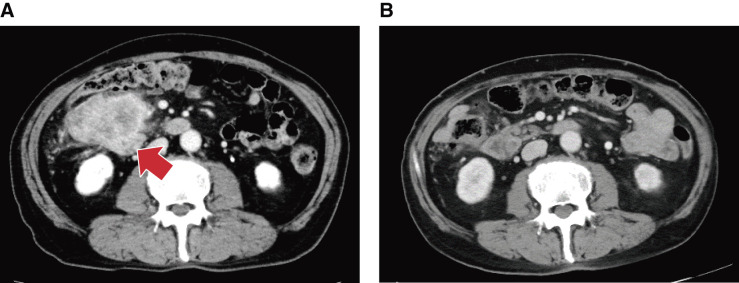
Comparison of pre- and post-immunotherapy on CT scan. (A) Duodenal invasion by the ascending colon cancer. The descending part of the duodenum is at the tip of the red arrow. (B) The tumor has shrunk significantly and a gap was formed between the tumor and the duodenum.
